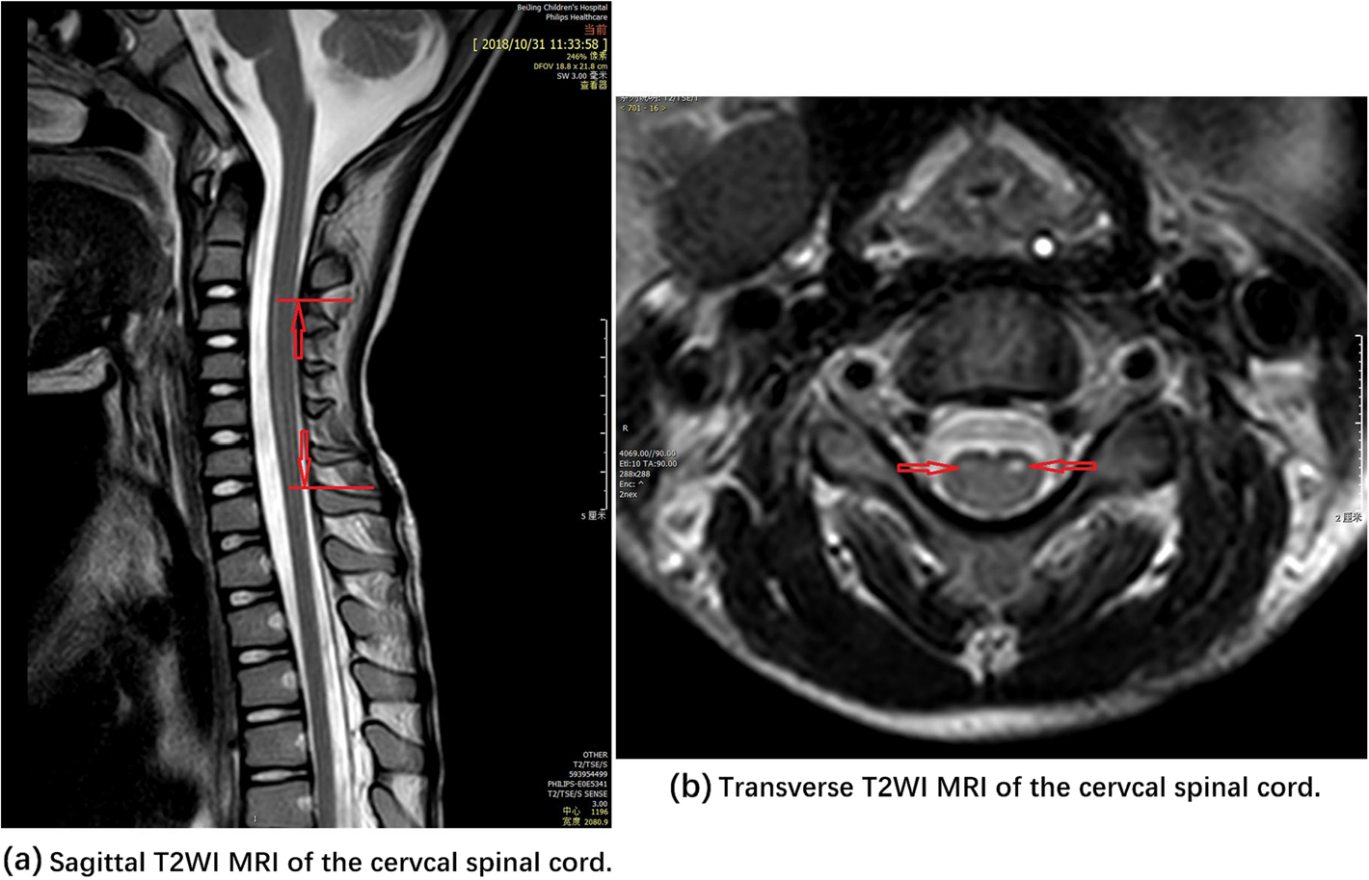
Sagittal and Transverse T2WI MRI of the cervical spinal cord. *Note: Arrows show abnormal signals of vertricornu over C2-C6. MRI = magnetic resonance imaging, T2WI = T2-weighted image
